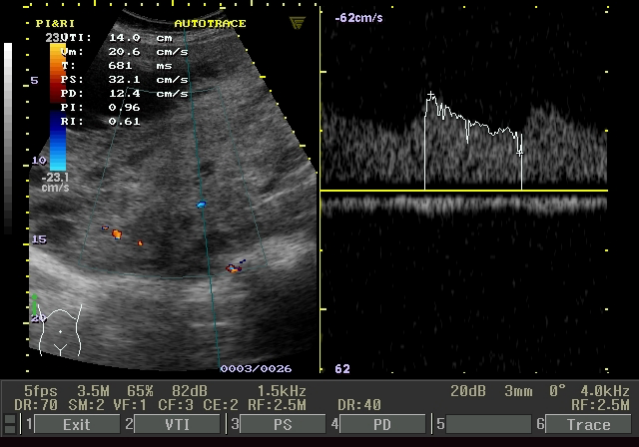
US and Doppler examination of the leiomyoma. US examination showing a heterogeneous echotexture of the mass and presence of multiple echo free areas, Doppler blood flow indices in and around the huge leiomyoma recorded a resistance index of 0.61 and pulsatility index of 0.96
